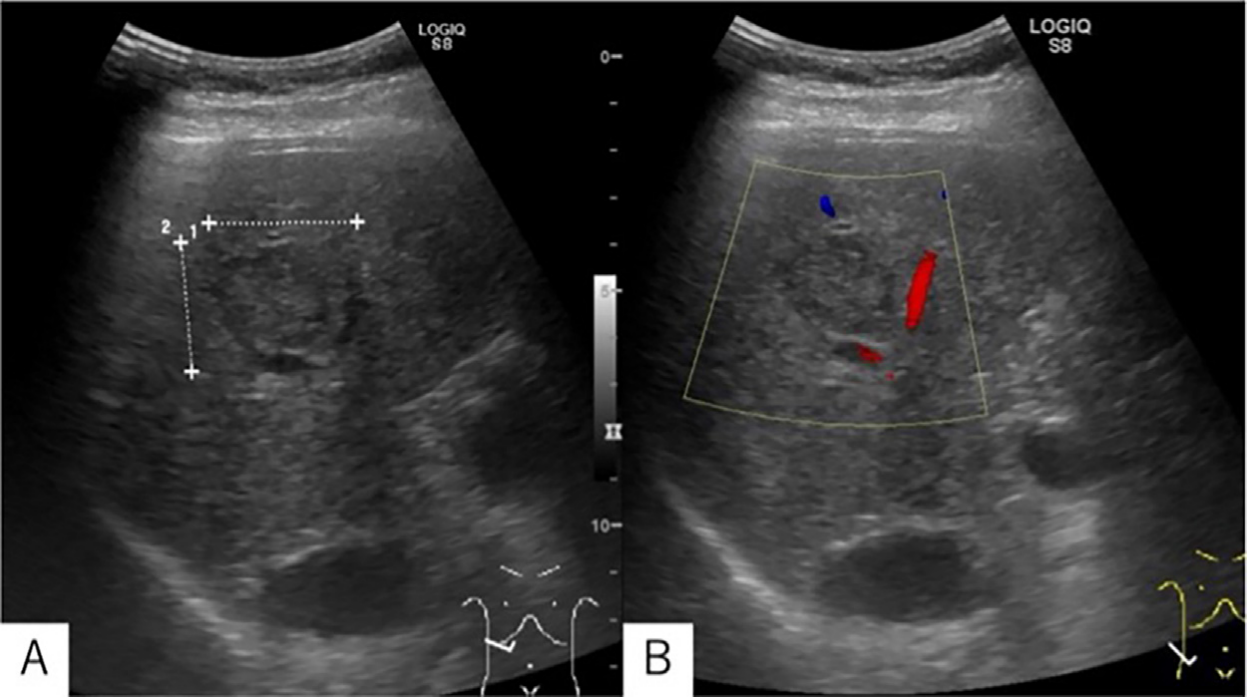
Abdominal ultrasound performed during a routine health checkup revealed a 3 cm mass-like lesion in the posterior segment of the right hepatic lobe. The lesion exhibited mixed echogenicity with both hyperechoic and hypoechoic areas but was overall isoechoic relative to the surrounding liver parenchyma. Posterior acoustic enhancement was observed. A peripheral band-like hypoechoic rim surrounded the lesion. No blood flow signals were observed in the lesion in Color Doppler, and no changes in echo intensity (chameleon sign) were observed with position changes (Not listed).
